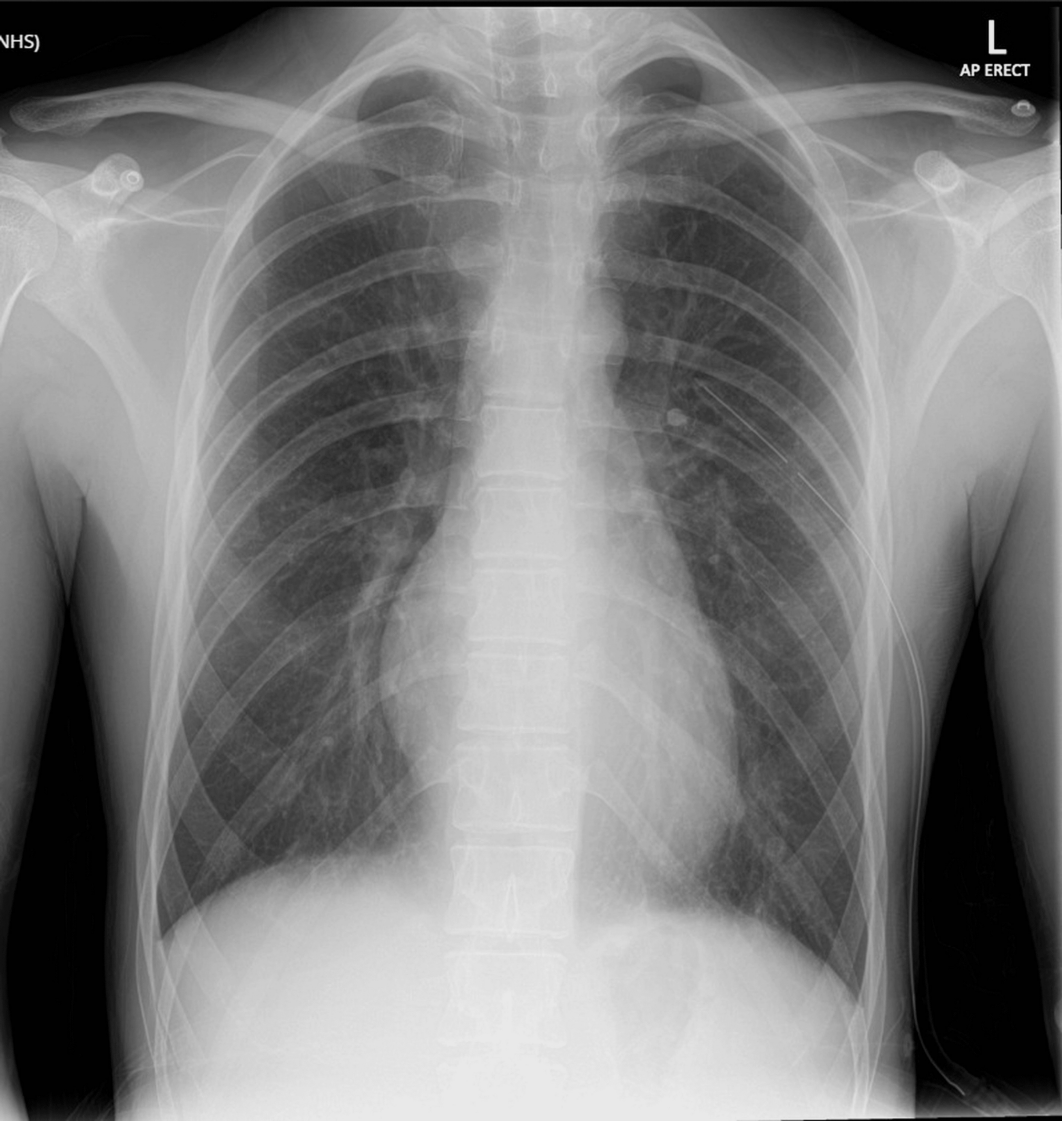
CXR after chest tube insertion demonstrating lung re-expansion and correct tube position. CXR, chest X-ray
